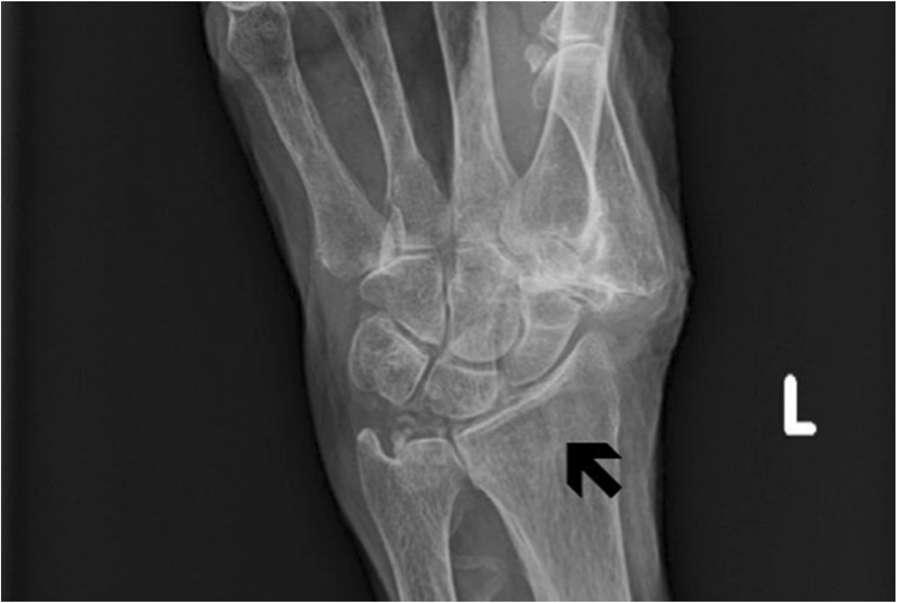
X-ray of the left wrist: a non-displaced fracture of the left distal radius (black arrow) can be seen.
